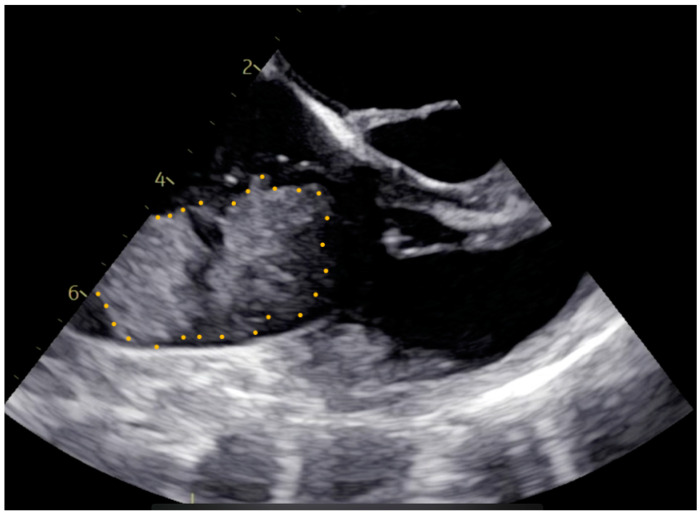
Pre-operative transesophageal echocardiogram image. The myxoma boundaries are delineated with a dotted yellow line.
